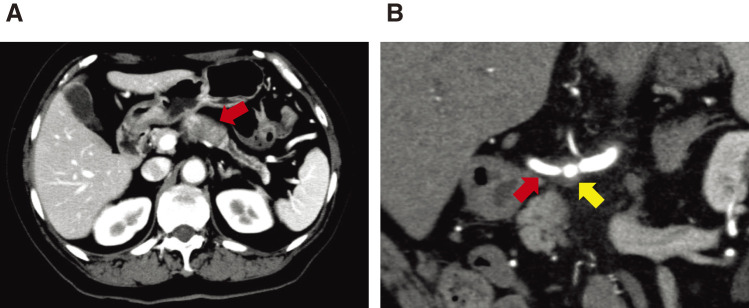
Radiological examinations before initial surgery. (A) Computed tomography (CT) showing a 43-mm hypovascular tumor in the pancreatic body (arrow). (B) The tumor contacts with the common hepatic artery (<180°) (red arrow), and the celiac artery (<180°) (yellow arrow).
